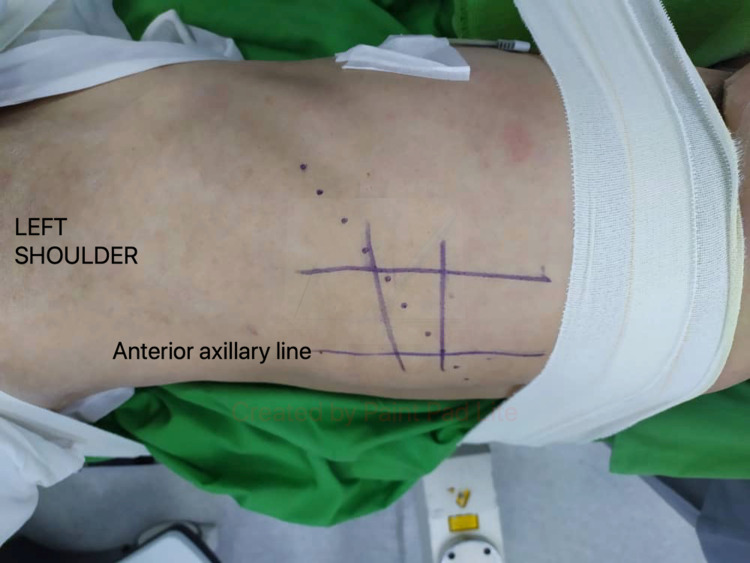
Surgical incision marking (dotted line) for transthoracic approach.
